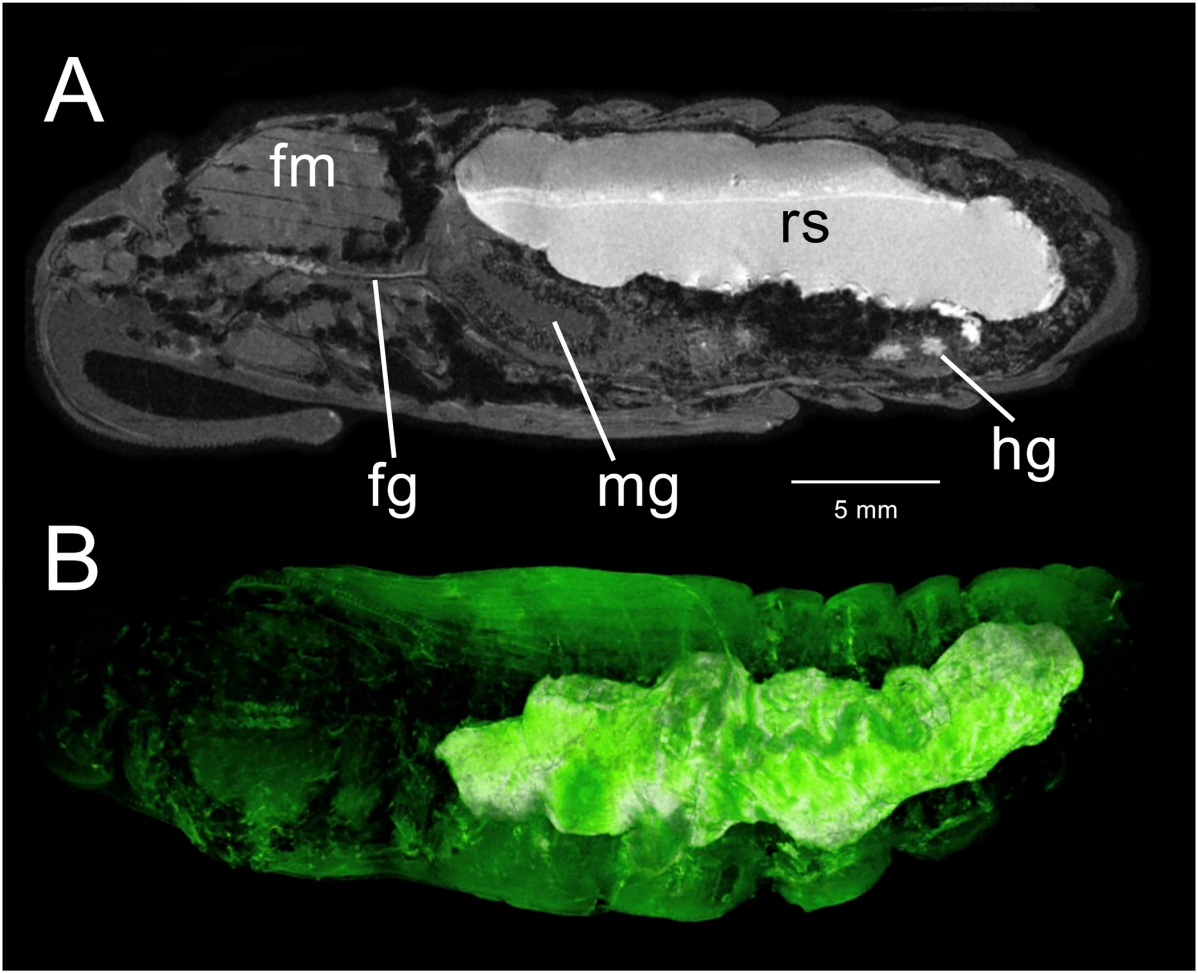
3D-GRE MR imaging data acquired from a *M*. *sexta* pupa 22–23 days after metamorphosis, approximately 12 hours before adult emergence. (A) Mid-sagittal view. The midgut (mg) is emptied of residual CA and the rectal sac (rs) spans the length of the abdomen. Flight muscles (fm) appear to be fully formed. Dark regions begin to appear in the thorax. (B) Ventral 3D surface-rendering showing the rectal sac and the outline of the anterior hindgut (hg). The midgut is not visible in this image.
